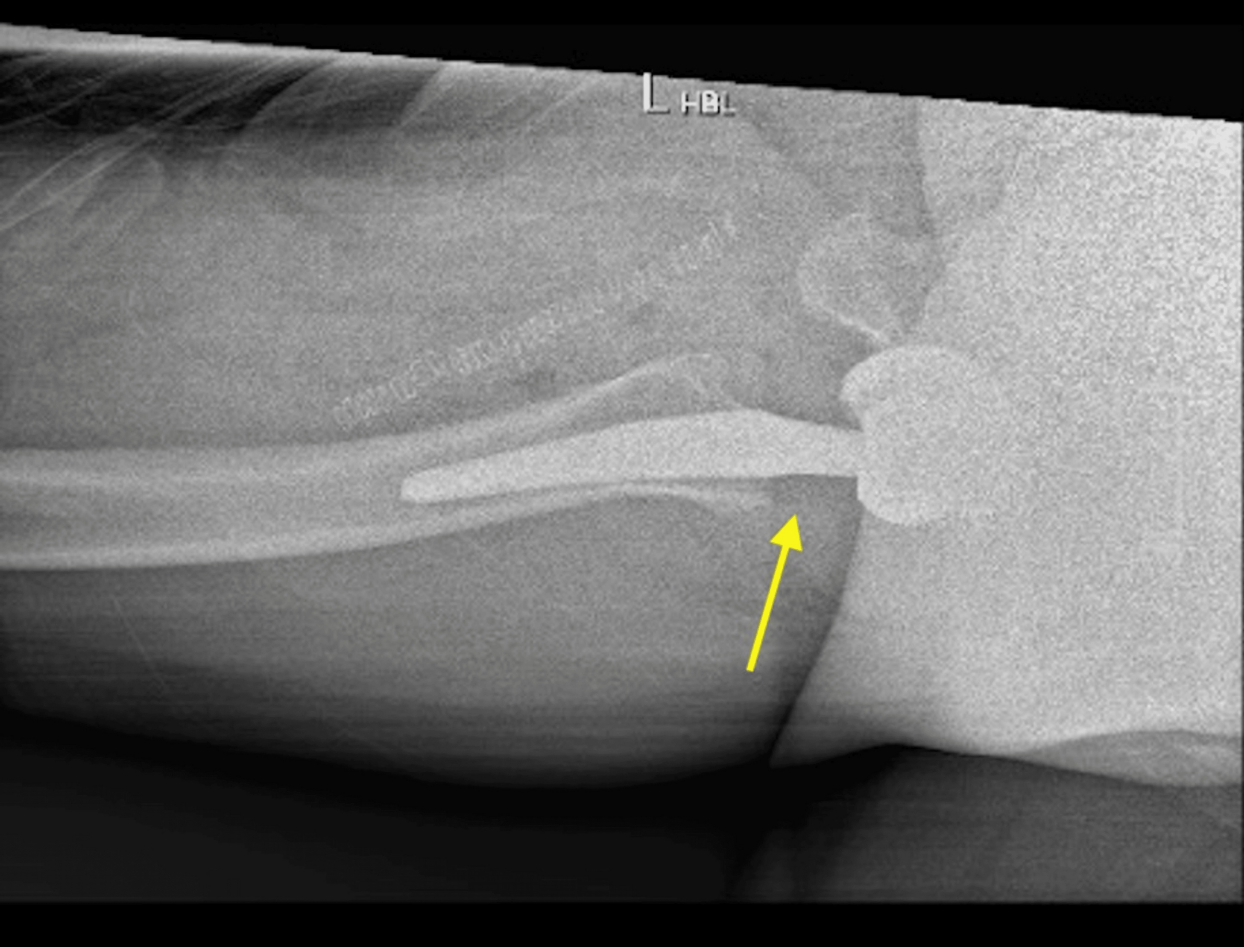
Post-operative lateral X-ray
